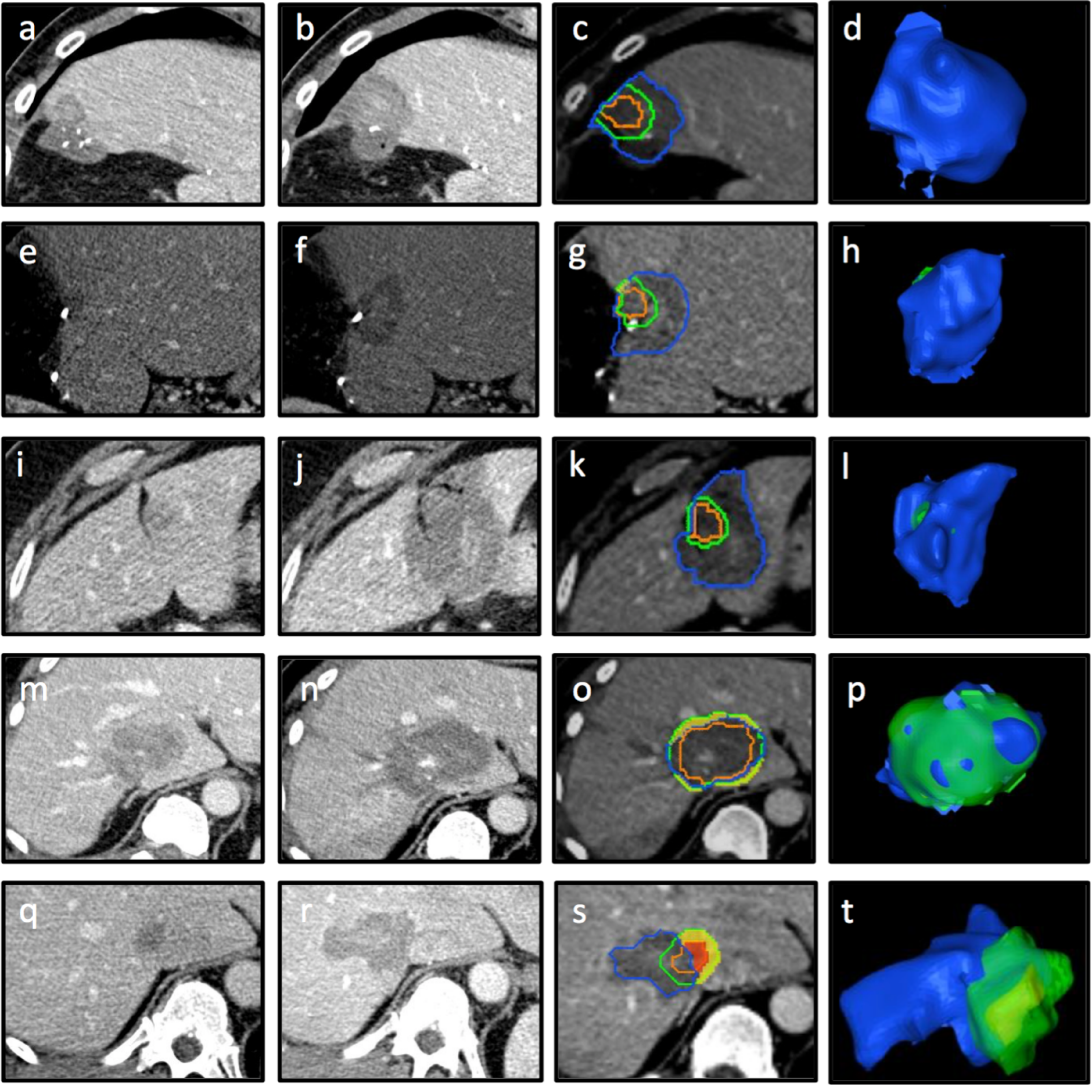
Examples of periablational 3D safety margin (SM) assessment with Ablation-fit^TM^. Complete ablation with 100% 3D SM (a–d), 95–100% 3D SM (e–h), 90–95% 3D SM (i–l), < 90% 3D SM (m–p), and incomplete ablation with residual tumor (q–t). a, e, i, m Pre-interventional CT-scan. b, f, j, n Post-interventional CT-scan. c, g, k, o Non-rigid registration of pre- and post-interventional CT scans with assessment of 3D SM of 5 mm: tumor (orange line), 5-mm safety margin (green line), necrosis zone (blue line), unablated safety margin (yellow area); unablated tumor (red area). d, h, l, p 3D reconstruction of necrosis zone (blue), unablated 3D safety margin (green), and unablated residual tumor (yellow)
